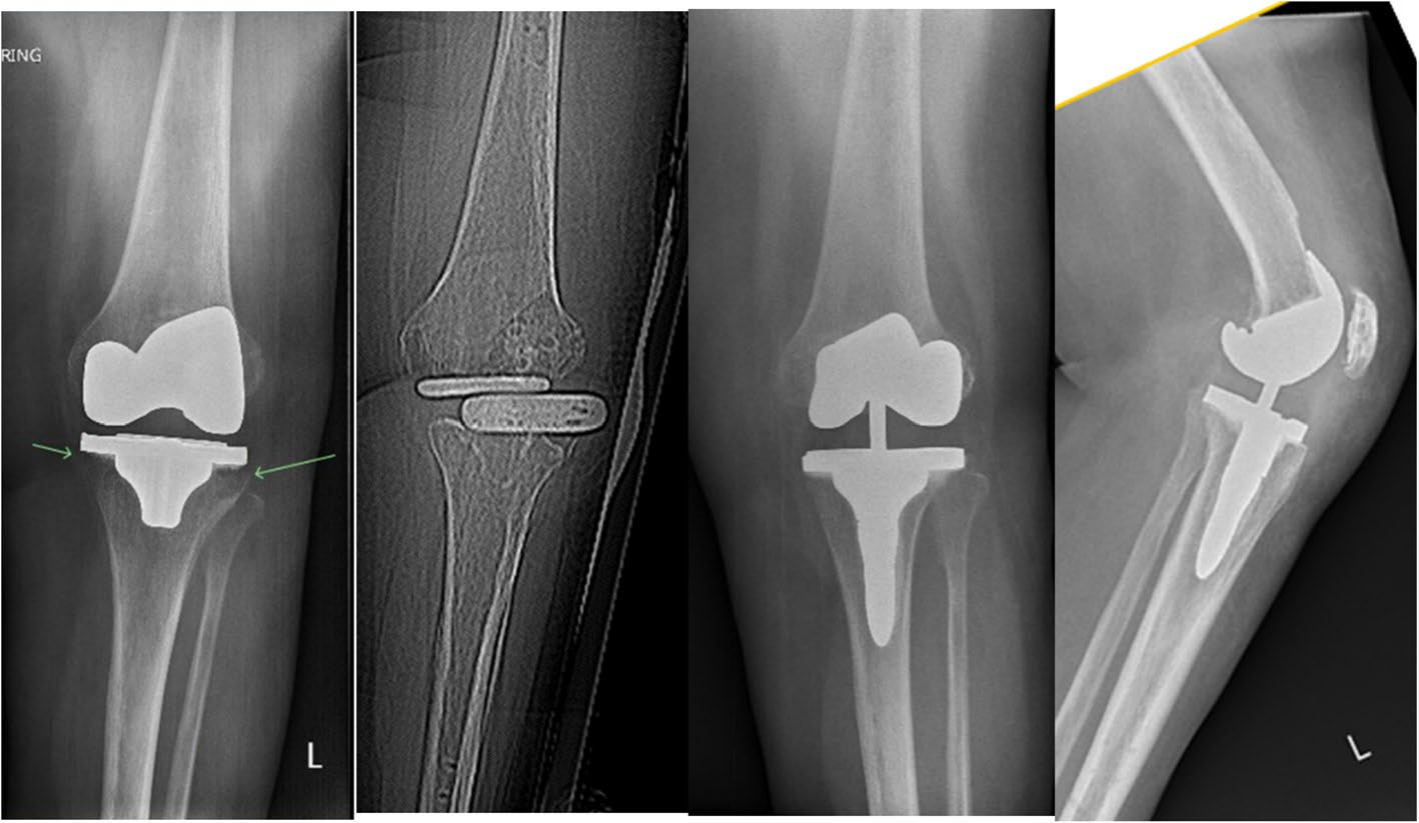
Pre- and postoperative radiographs of the patient for the preoperative planning in Fig. 15
